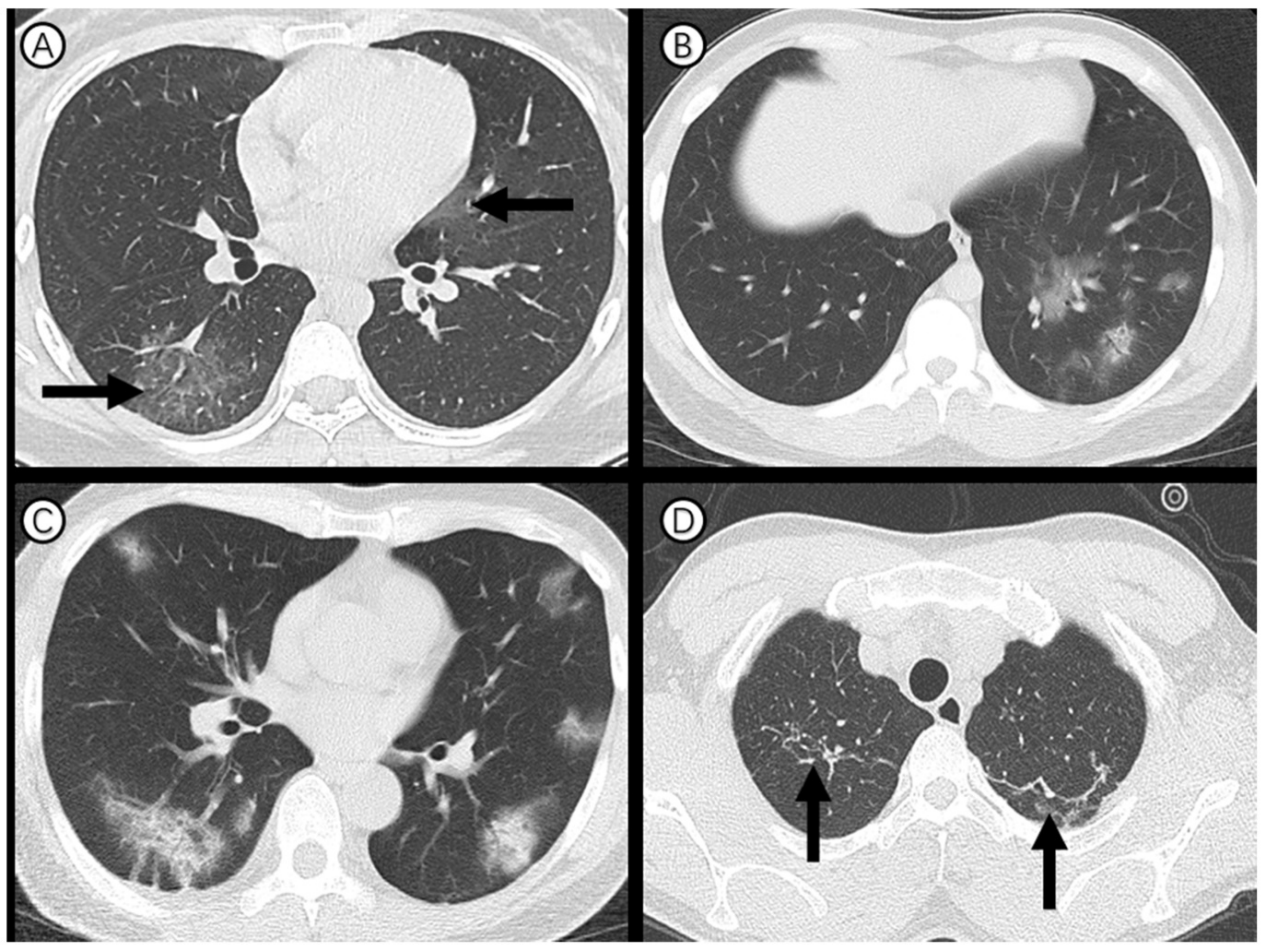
Axial thin-section CT scans in medical staff infected with SARS-CoV-2. (A) 27-year-old woman: bilateral, peripheral ground-glass opacity in the right lower lobe and left upper lobe (arrow). (B) 27-year-old man: unilateral, multiple and perbronchovasular ground-glass opacity associated with air bronchograms in the left lower lobe. (C) 55-year-old man: bilateral, peripheral ground-glass opacity mixed consolidation pattern. (D) 28-year-old man: bilateral and linear atelectasis in the right and left upper lobes (arrow), regarded as chronic lung lesion with lack of changes on serial CT examinations.
